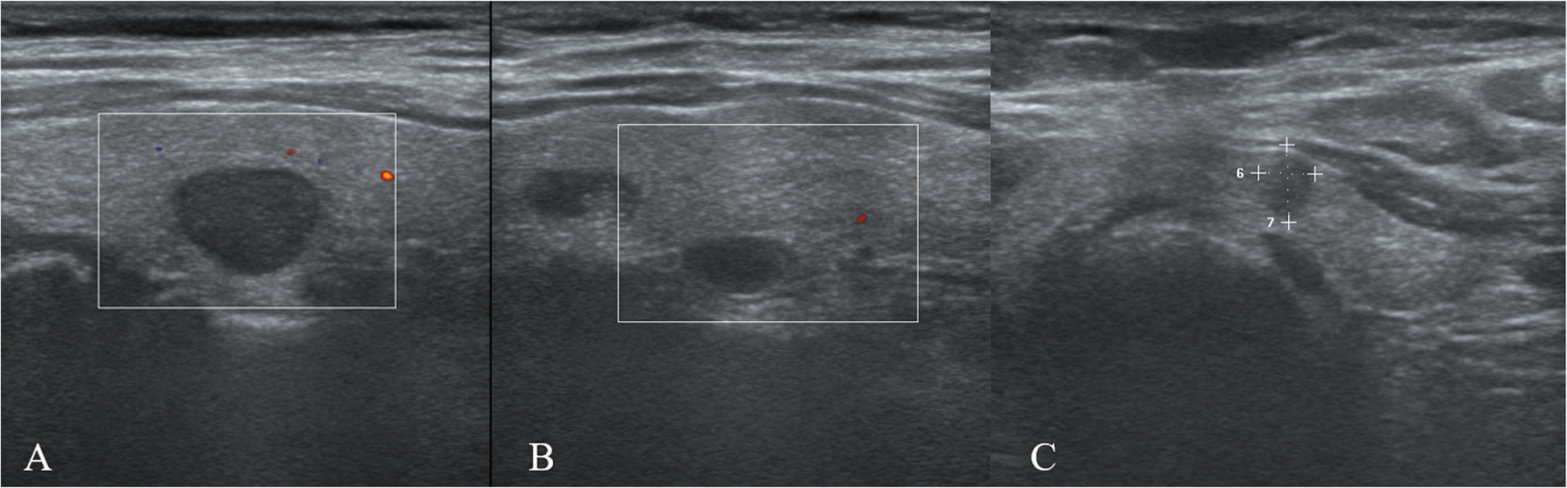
Ultrasonography image of hypoechoic nodules with well-defined margins located in the right inferior lobe of the thyroid gland (a). A typically cystic parathyroid adenoma with homogeneously hypoechoic and an elongated shape was located in posterior left superior thyroid (b). Ultrasonography showed a hypoechoic nodule with irregular margins with a taller-than-wide shape located in the left superior thyroid, which was confirmed as papillary carcinoma by FNAB (c)
